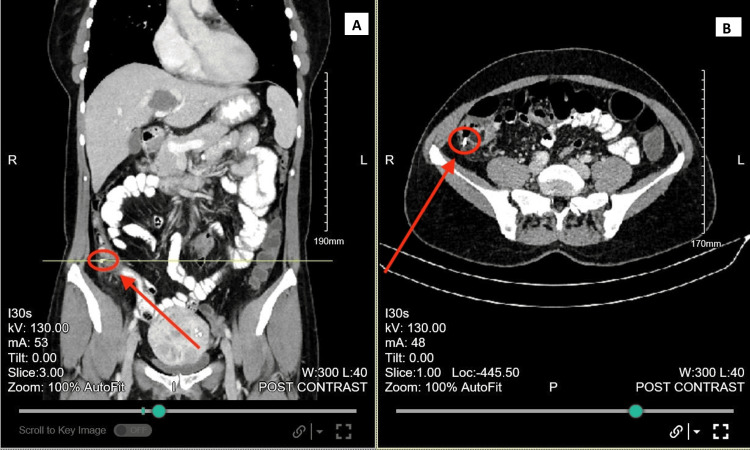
Computed tomography (CT) scan showing an appendiceal mucocele (coronal view on left and axial view on right).
